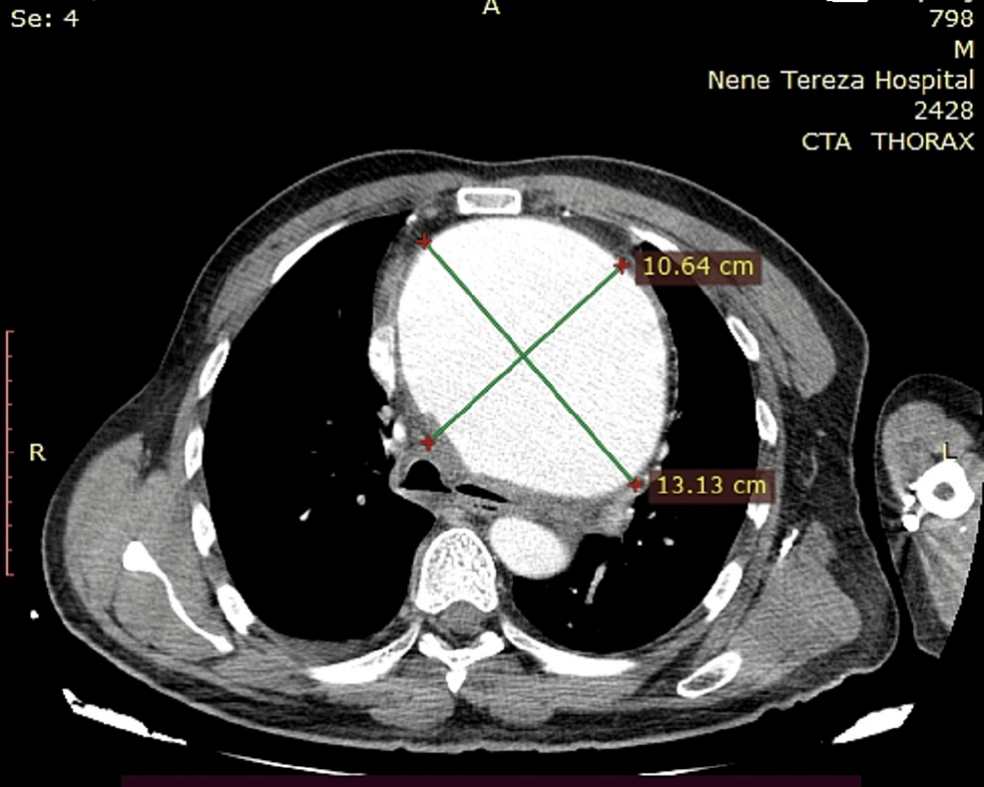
Computed tomography with intravenous contrast The maximal aortic aneurysm dimensions are depicted in the image.
